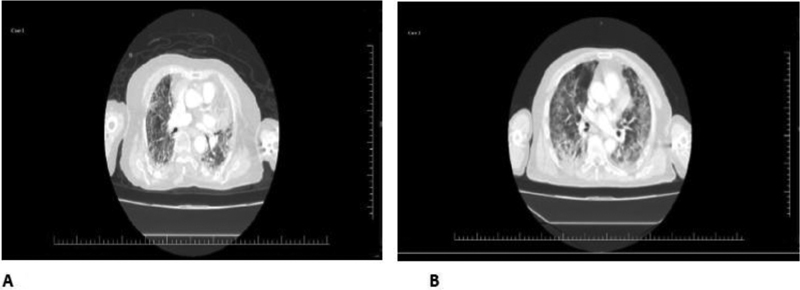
Views from chest computed tomography of the cases (
